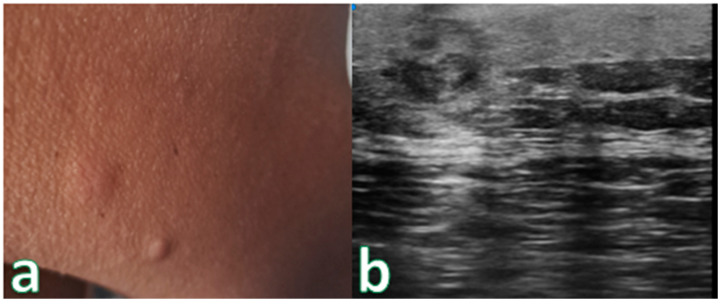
(a) Nodular skin lesion without signs of flogisis in the right deltoid region. (b) Exam performed with a very high-frequency ultrasound probe at 50 MHz: Iso-hypoechoic lesion with irregular and hyperechoic margins infiltrating the epidermis and the subepidermal layer. Pathologic Assessment revealed B-Cell Lymphoma.
